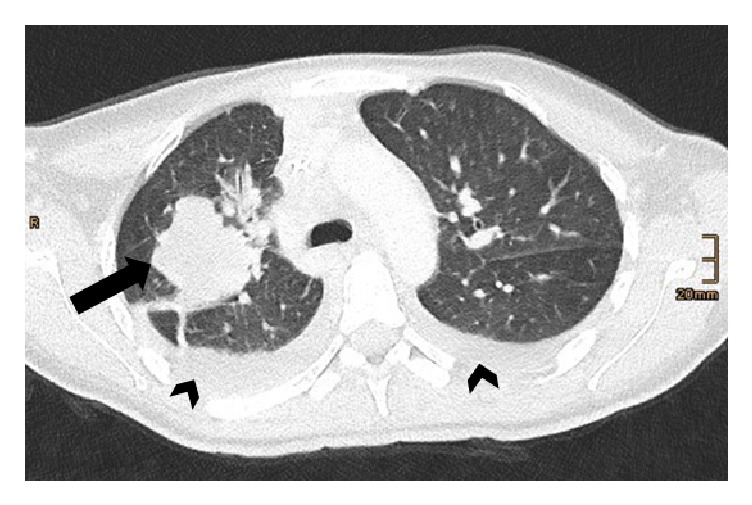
Chest computed tomography (CT) scan demonstrates a mass lesion (5 cm) in the right upper lobe of lung (arrow), and bilateral pleural effusions (arrowheads).
